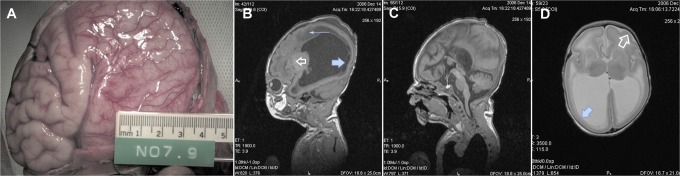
Brain pathology and MRI findings in XLAG: (A) cerebrum at autopsy reveals posterior lissencephaly with transition to anterior pachygyria, (B) sagittal T1 MR sequence demonstrates lissencephaly P>A and marked lateral ventriculomegaly. Note subependymal cysts (large arrow) abnormal periventricular white matter signal (small arrow) and abnormal basal ganglia signal clear arrow, (C) sagittal T1 MR sequence of the midline demonstrates agenesis of the corpus callosum, (D) axial T2 MR sequence demonstrates colpocephaly and lissencephaly with transition from posterior agyria (solid arrow) to anterior pachygyria (open arrow). MR indicates magnetic resonance; XLAG, X-linked lissencephaly with abnormal genitalia.
